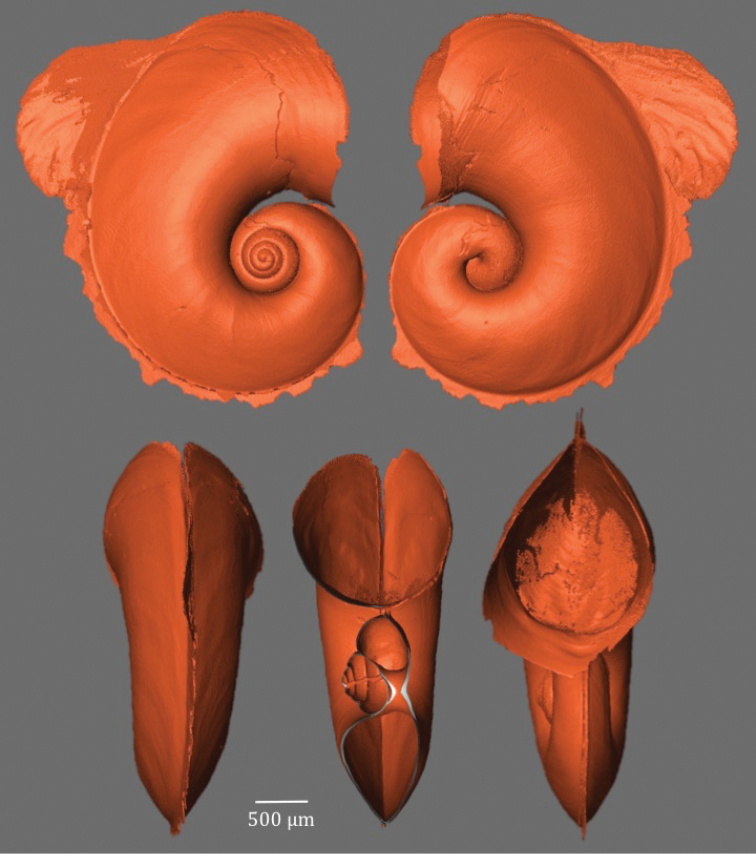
X-ray tomography of *Atlanta
